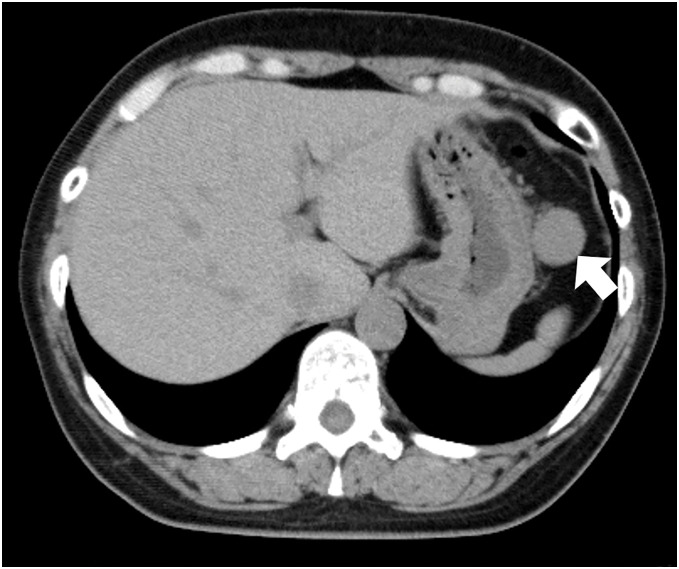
CT. Tumor near the upper part of the gastric body. The continuity with the stomach was not clear.
